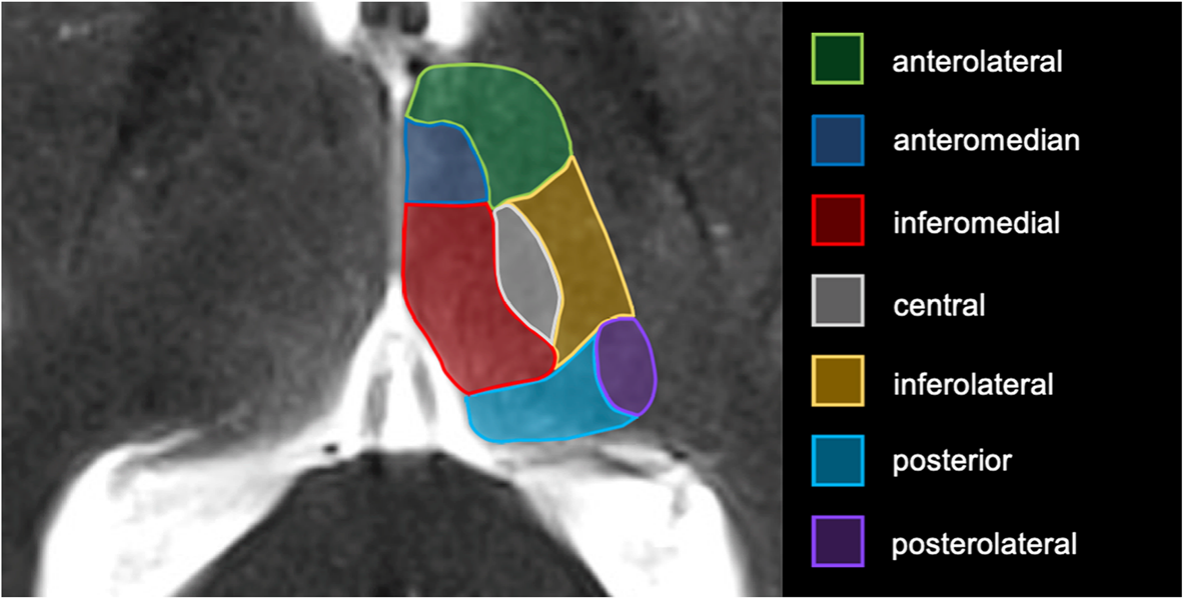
The thalamic vascular territories as defined in this study projected on an axial T2-weighted MRI template. The map was created based on the literature [4, 7, 9, 12, 22] and includes the four traditional and three variant type vascular territories
